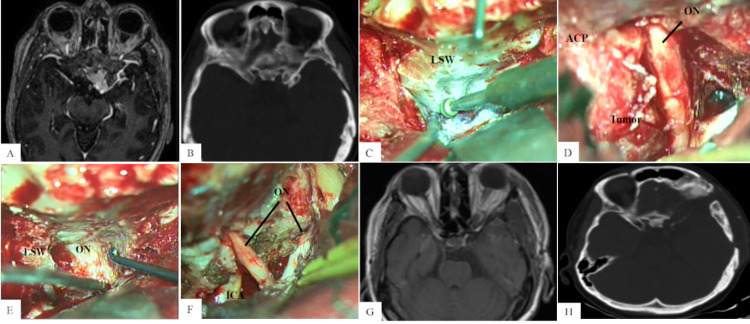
Hybrid technique for complete AC (A) Preoperative contrast-enhanced axial T1-weighted MRI shows a complex type II anterior clinoidal meningioma on the left side extending into the optic canal. (B) Preoperative axial bone window CT scan shows large hyperostosis of the ACP. (C) First step: extradural drilling of the sphenoid ridge. (D) Second step: intradural exploration showing the optic nerve compressed by the tumor and the hyperostotic ACP. (E) Third step: proceeding with total extradural AC. (F) Gross total resection of the tumor and decompression of the optic nerve is achieved. (G) Postoperative contrast-enhanced axial T1-weighted MRI shows no residual tumor. (H) Postoperative axial bone window CT scan shows total AC. AC, anterior clinoidectomy; LSW, lesser sphenoid wing; ACP, anterior clinoid process; ON, optic nerve; ICA, internal carotid artery
